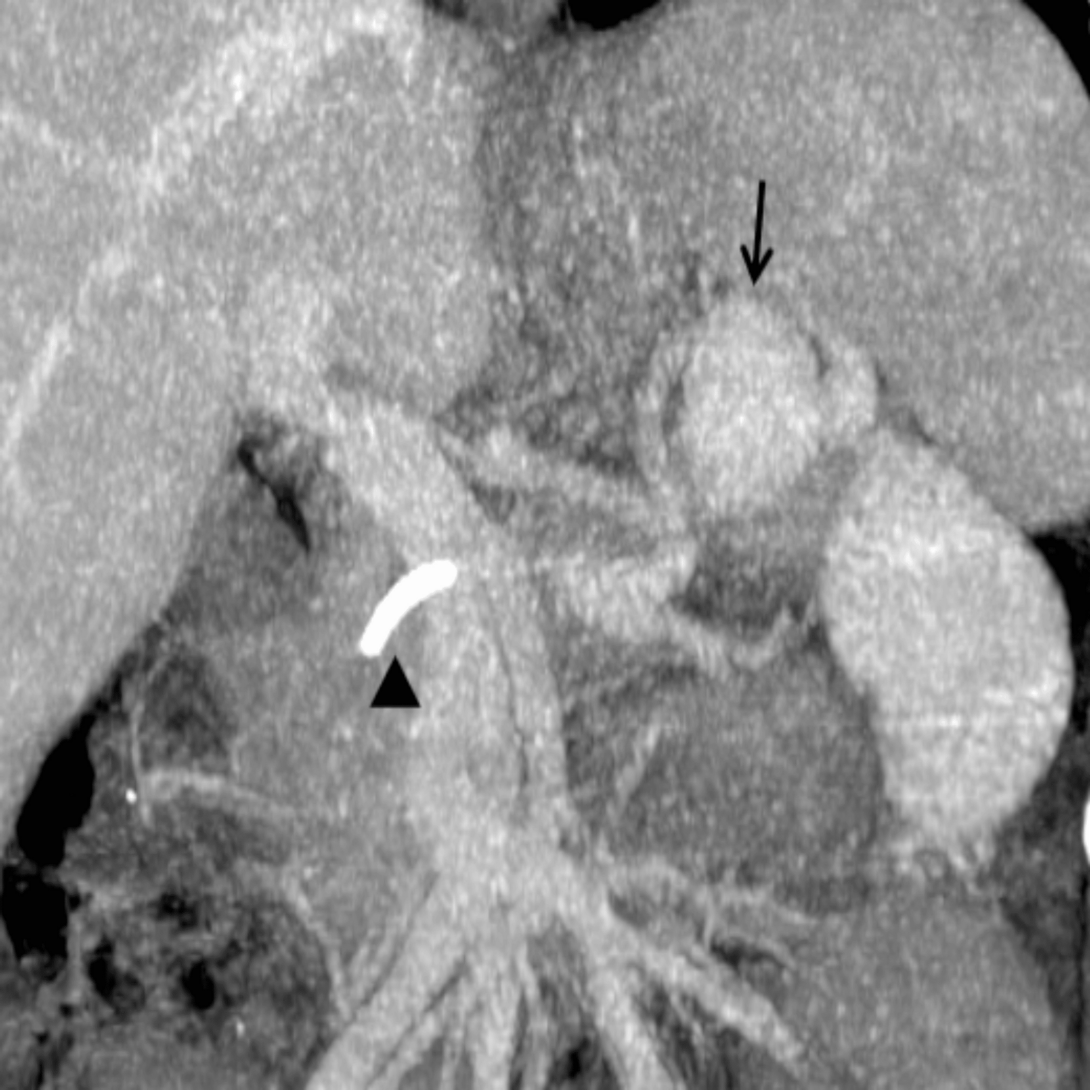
Coronal reconstructed maximum intensity projection (MIP) image from the venous phase of a contrast-enhanced CT abdomen shows the splenic vein varix (arrow). A stent is visible within the main pancreatic duct (arrowhead).
